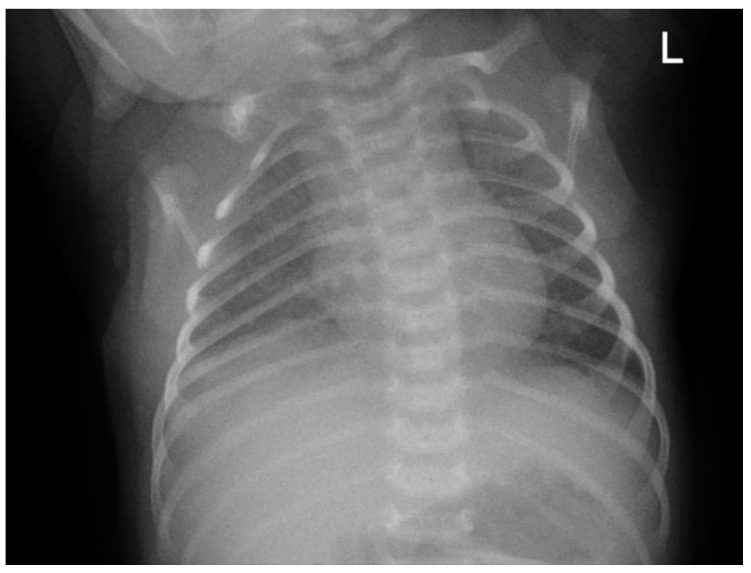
Anteroposterior (AP) chest X-ray on day 1 of admission to the emergency department (ED) showing normal cardiac and pulmonary structures with no abnormalities detected. “L”: an anatomical marker indicating the left side.
